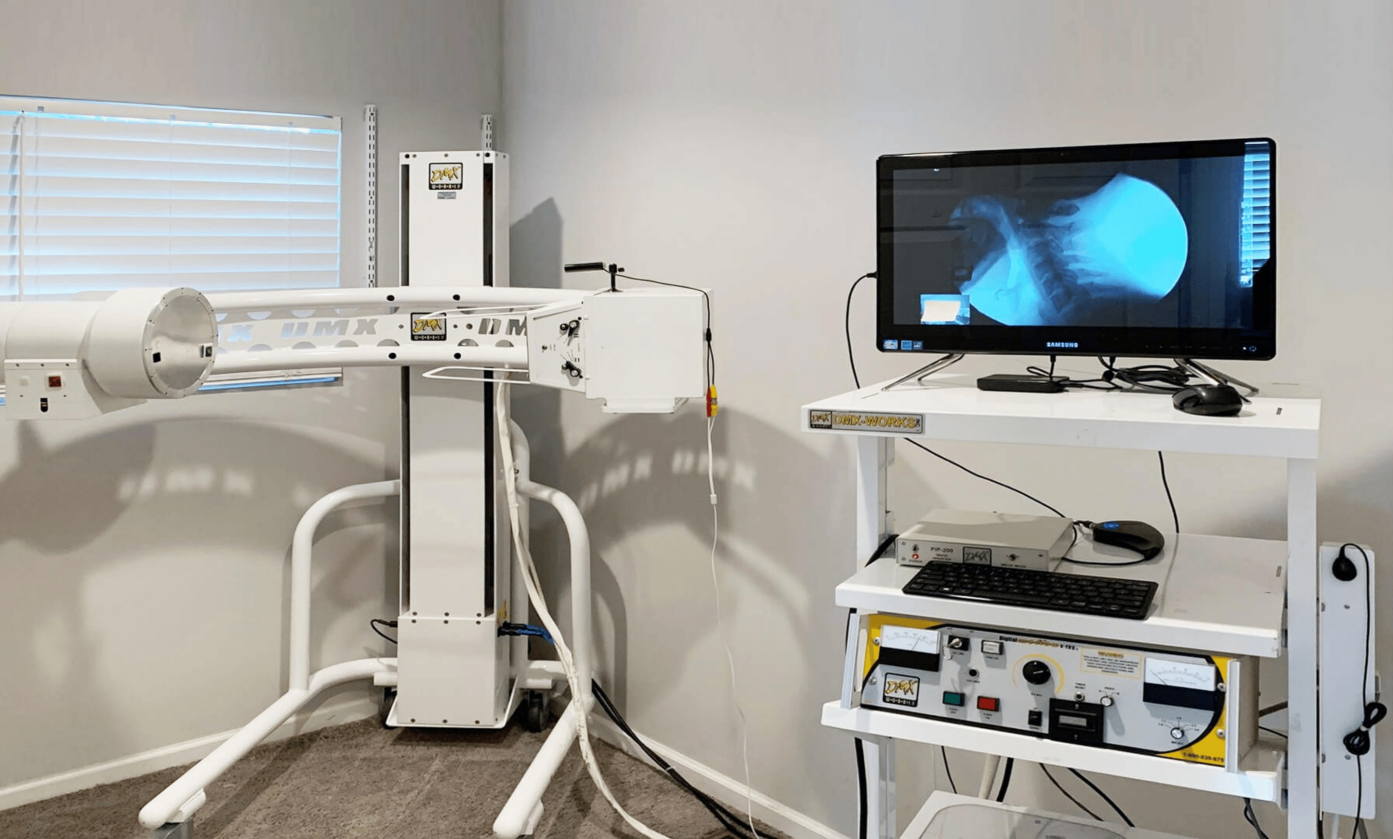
DMX Digital Motion X-ray equipment The equipment emits X-rays in real time to capture the cervical spine while the patient is put through various ranges of motion. Source: Used with permission from DMX Imaging, LLC (Palm Harbor, FL, USA).
